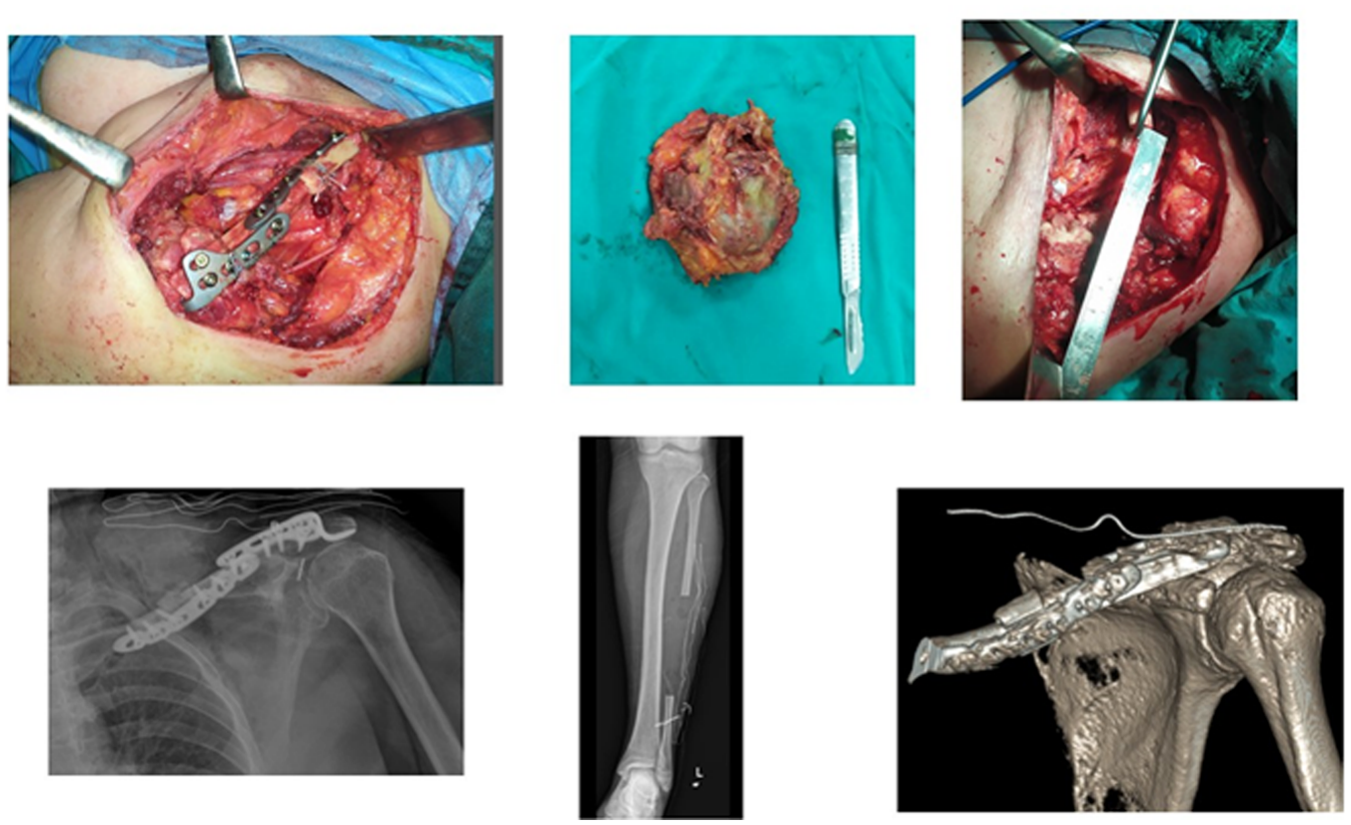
(Top) Images of an intraoperatively removed mass and fixation of a fibular graft endobutton. (Bottom) Perioperative direct radiograph and CT 3D.
